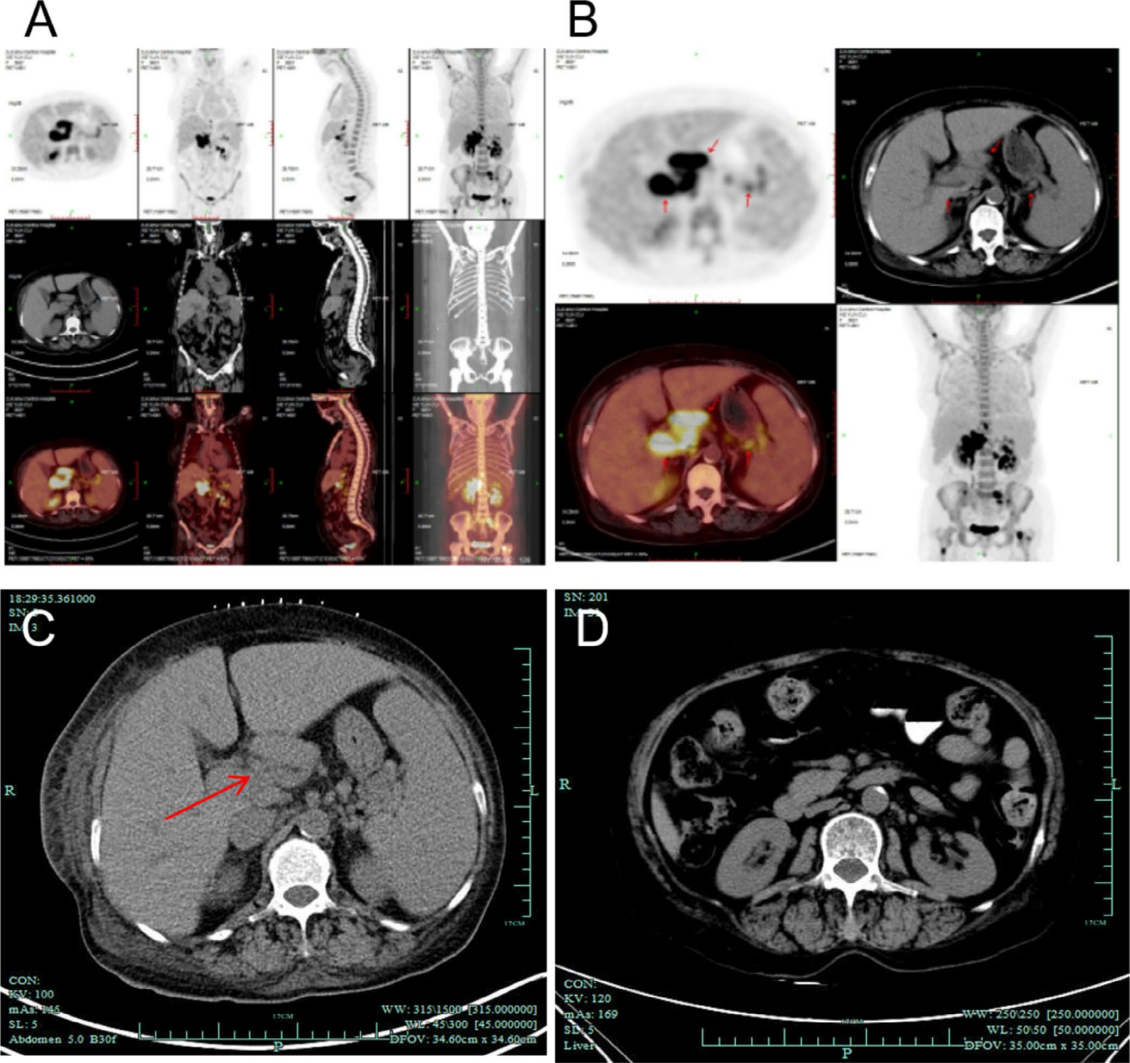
(A, B) PET–CT examination revealed multiple enlarged lymph nodes around the inferior vena cava, hepatic hilum, retroperitoneum and splenic hilum, as well as increased FDG metabolism. (C, D) The size of lymph nodes in abdominal CT was changed before and after R‐CHOP treatment.
